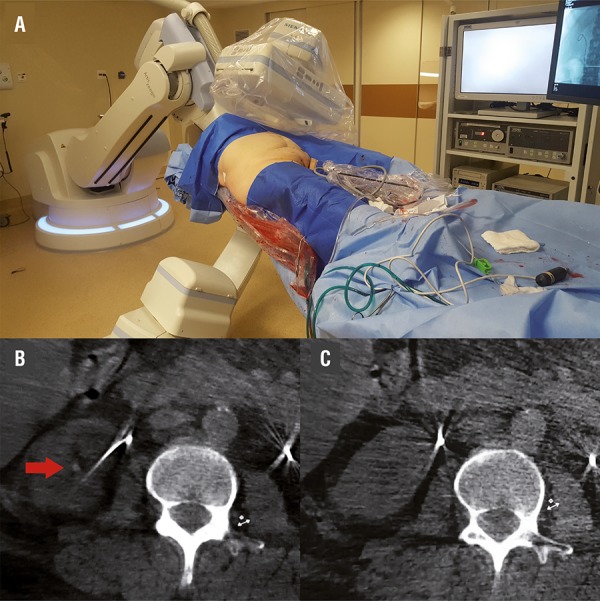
A) Intraoperative image acquisition by Artis Zeego – Uro Dyna-CT; B) Intraoperative CT scan showing one residual stone (arrow); C) intraoperative CT showing a stone free right kidney.
